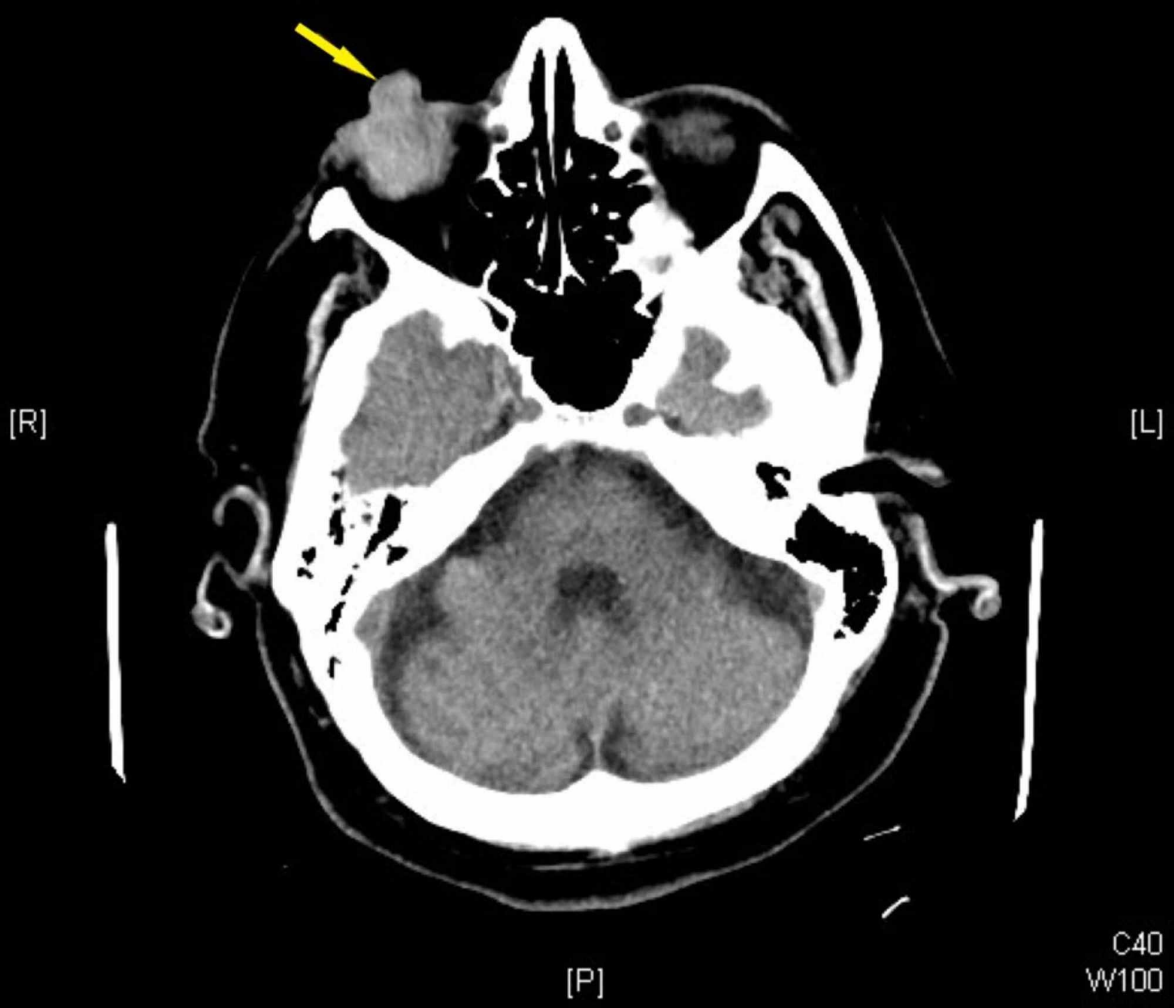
CT demonstrating extrusion of eye contents from right globe (arrow).
